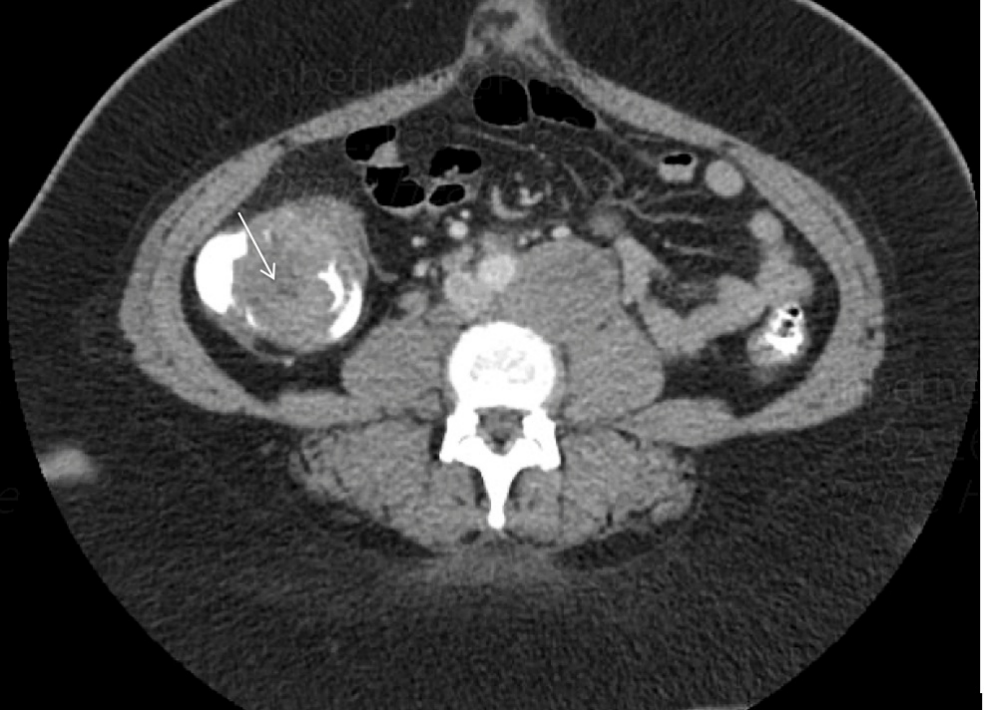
Axial computed tomography showing ascending colonic mass (white arrow)
